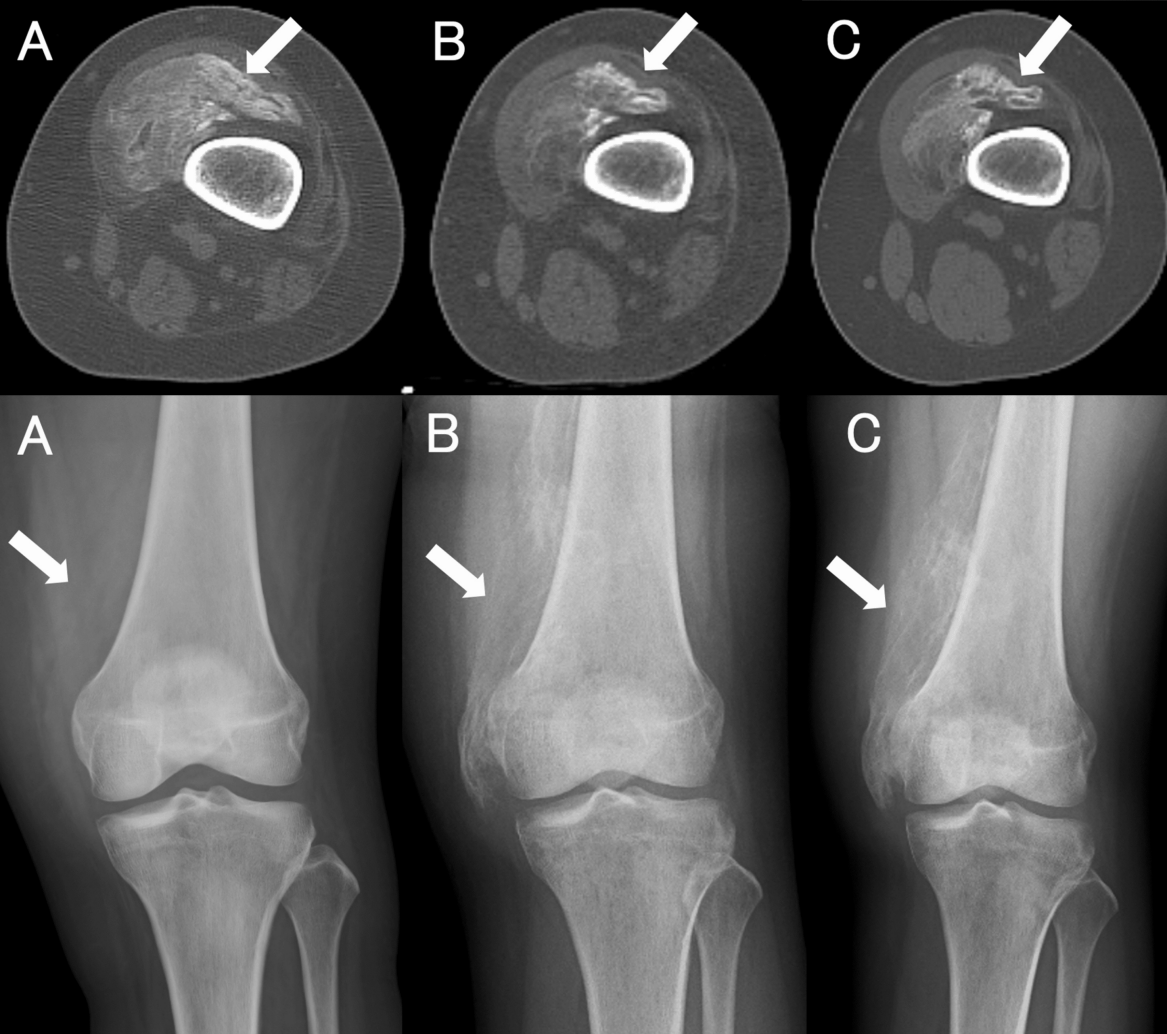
Changes over time in the left femur computed tomography scan (top panel) and X-ray image (bottom panel). A: Onset, B: Two months later, C: Four months later. The soft-tissue ossification shows well-developed cortex and medullary spaces (arrows).
